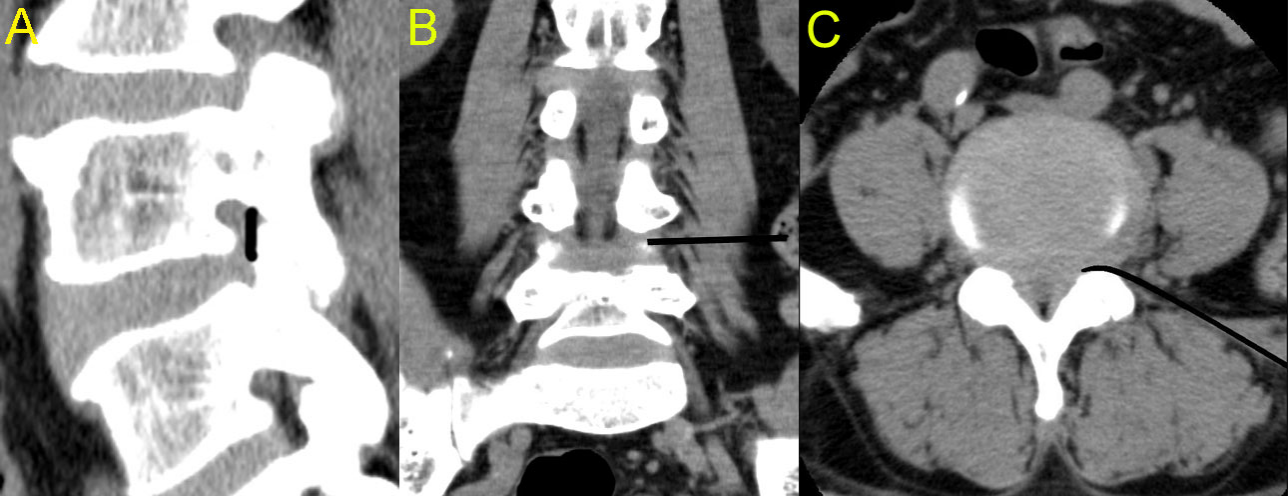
Three-dimensional CT images of the transforaminal epidural dry needling procedure. The sagittal CT image shows the area where the transforaminal epidural dry needling was performed (A). The coronal (B) and axial (C) CT images show the depth and route of needle insertion.
